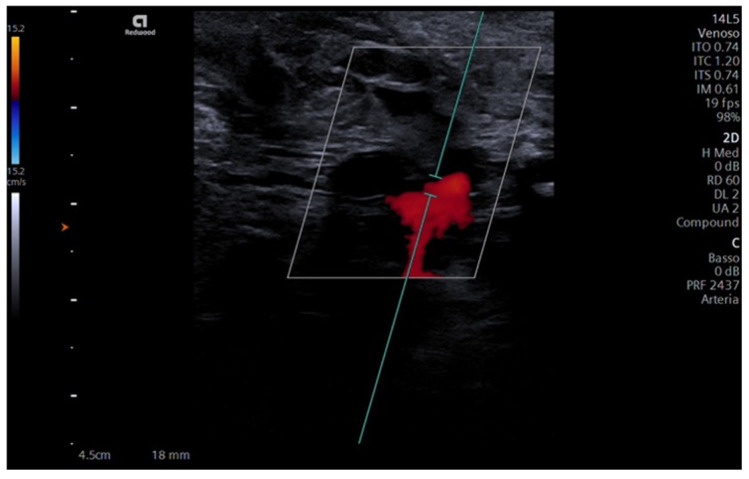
Doppler ultrasound. The figure shows the Color-Doppler ultrasound evidencing blood-mimicking fluid presence in the anastomosis between superficial temporal and facial arteries.
